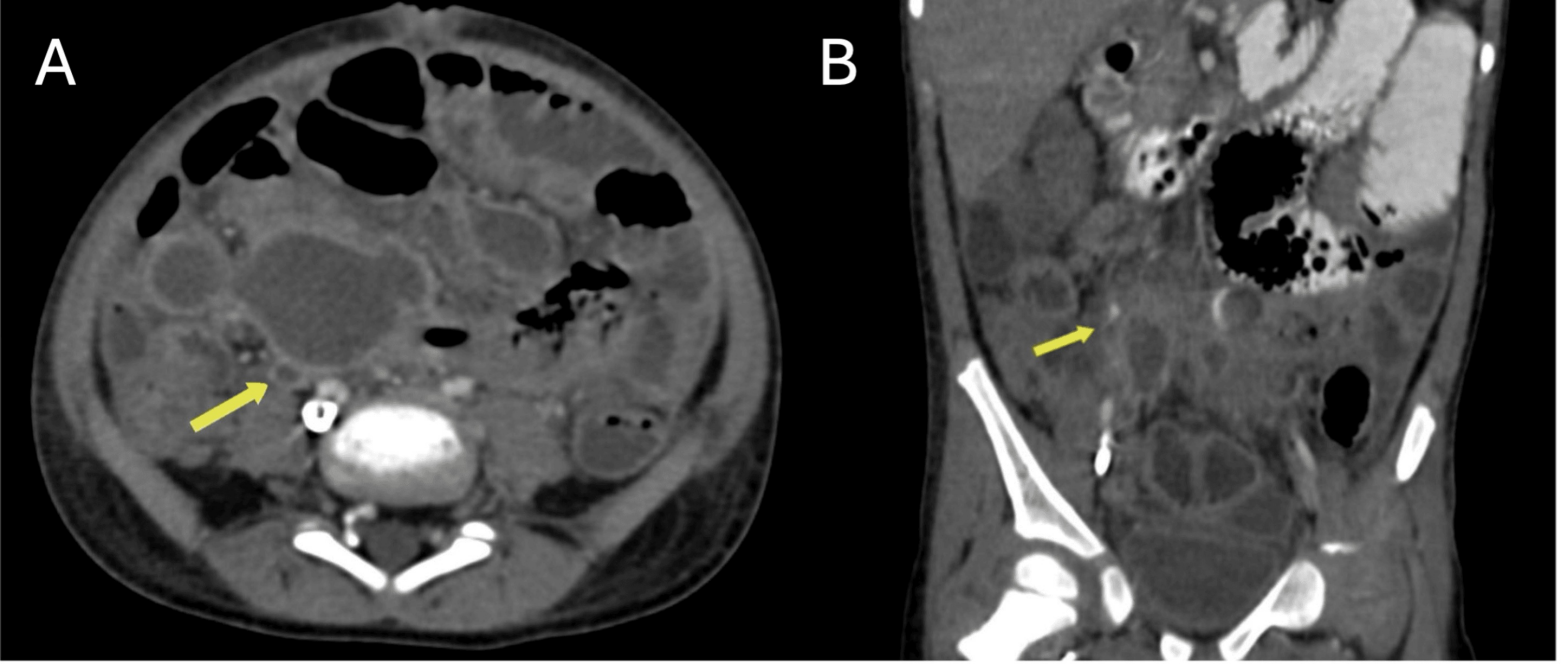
CT of a 13-year-old male with right iliac fossa pain. (A) Axial CT shows a hyper-dense appendicolith. (B) Coronal CT shows a dilated tubular structure in the right iliac fossa with surrounding inflammatory changes, consistent with appendicitis. CT: computed tomography
